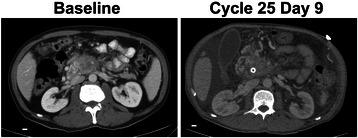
CT scans of pancreatic cancer patient. CT scan imaging at baseline (left) and at time of biopsy (right) demonstrates stability of hypovascular pancreatic mass. White bar indicates 1 cm
